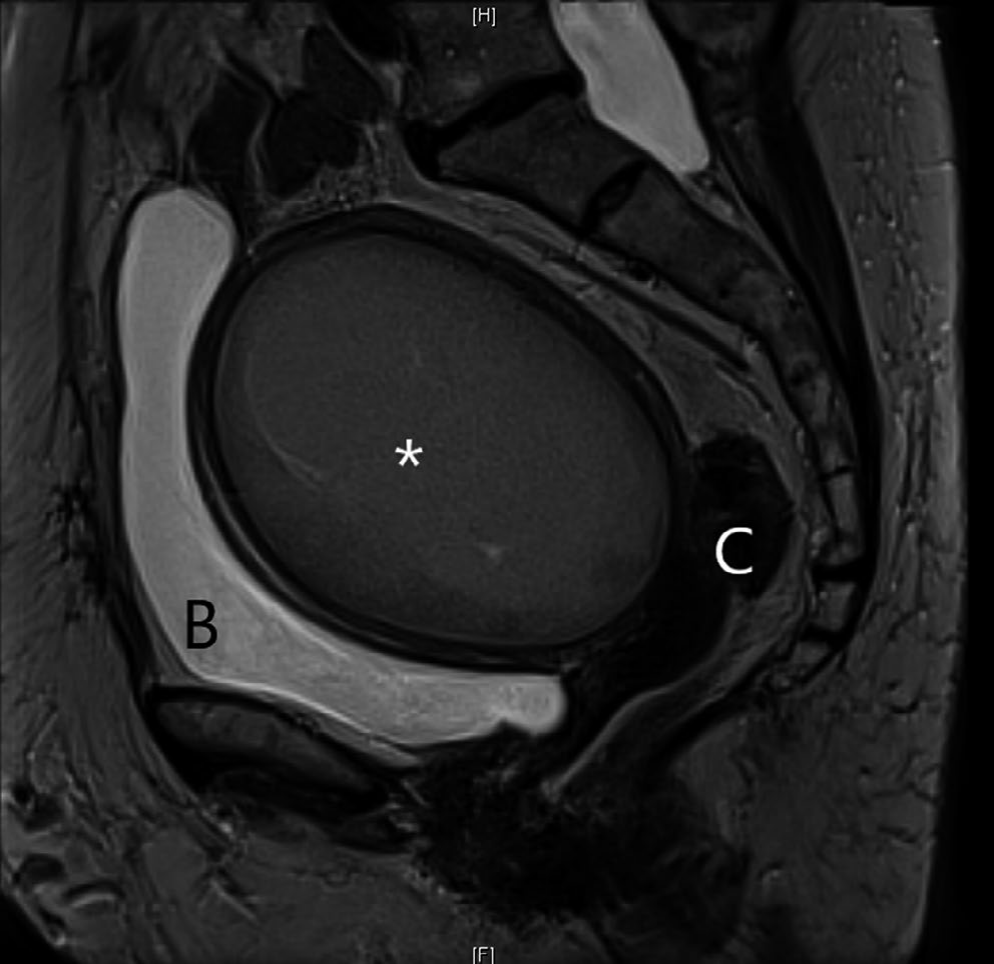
Magnetic resonance imaging of the pelvis (T2 sequence) revealed an enlarged uterus containing an intrauterine cystic mass (105 mm length), suggesting a pyometra (*). B, bladder; C, uterine cervix
